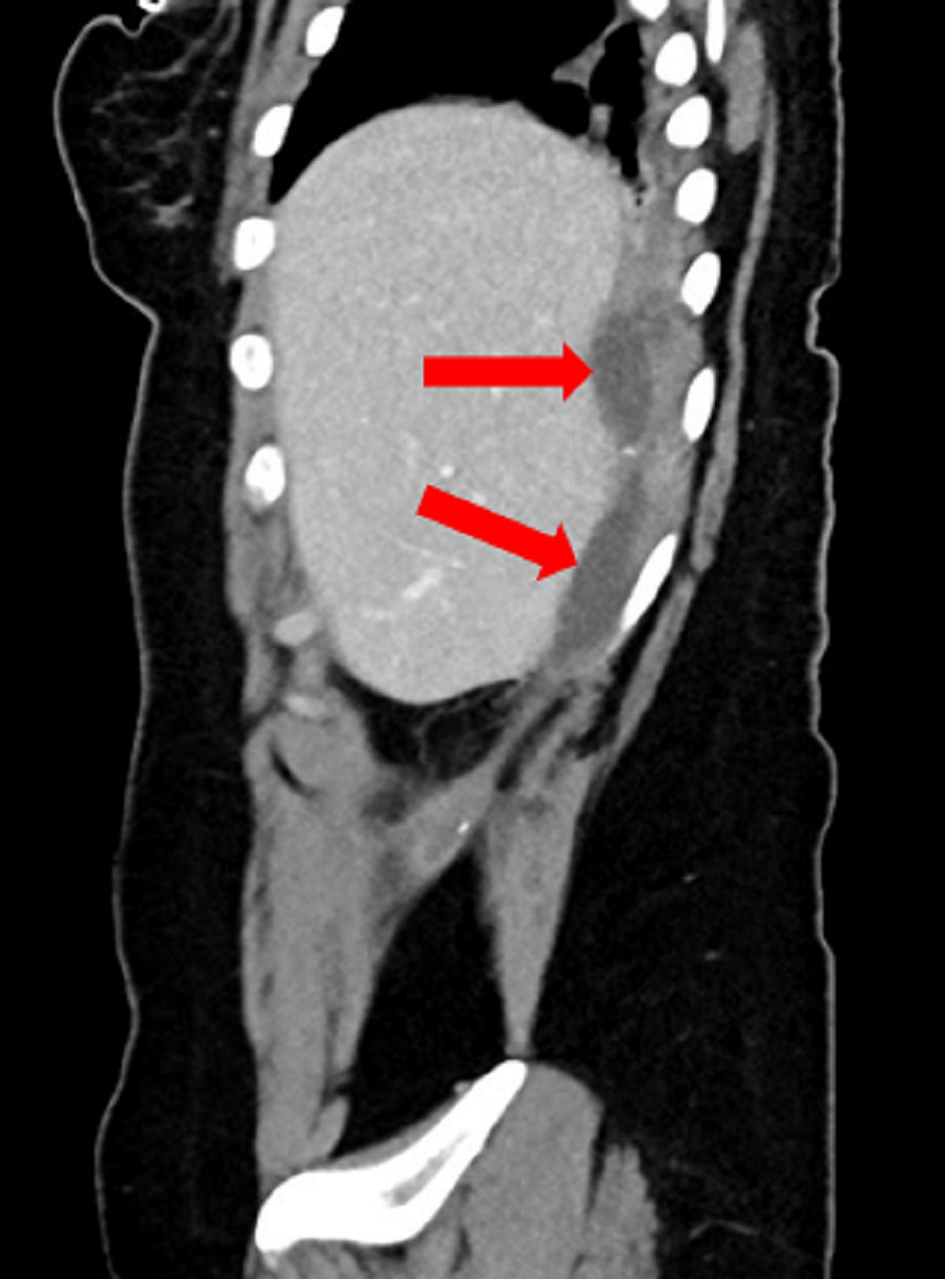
A sagittal view of the CT scan showing a subdiaphragmatic collection (red arrows). CT: Computed tomography.
